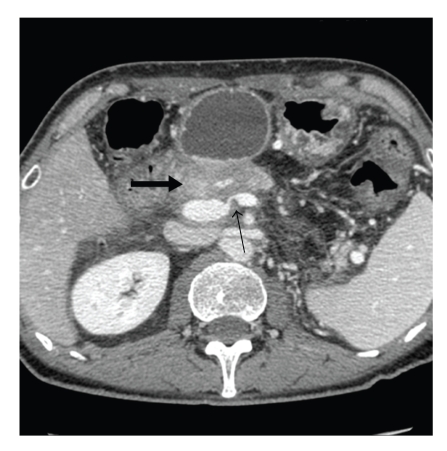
CT evidence of the recurrent pancreatic mass in the previous surgical bed (large arrow) and demonstration of obstruction of the splenic vein at the portal vein confluence (small arrow).
